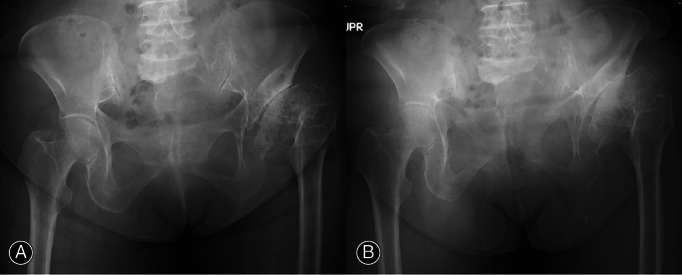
X‐ray of a patient with pelvic tilt and left hip osteoarthritis. Pelvic radiographs were taken in the standing and supine positions, and the pelvic tilt angle was calculated using the conversion formula described by Doiguchi *et al*.^13^ In this patient, the pelvic tilt angle was 48° in the supine position (A) and 55° in the standing position (B), with 7° of posterior pelvic tilt occurring during the postural transition
